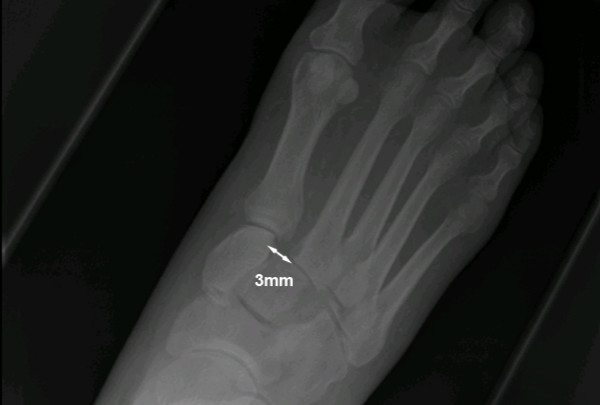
Dorsoplantar weight-bearing film of the right foot. The radiograph shows a 3 mm lateral displacement of the 2^nd ^metatarsal and a mild 2 mm lateral displacement of the first metatarsal consistent with a homolateral (Type B2) Lisfranc injury.
